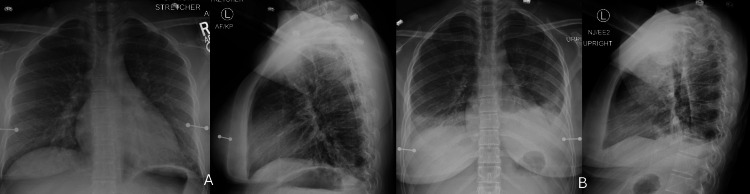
Chest radiographs on admission 3/25/21 (A) showing retrocardiac opacity and 3/28/21 (B) showing worsening bilateral consolidation
